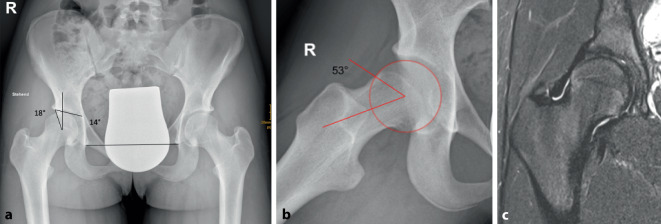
Die Cam-Morphologie kann bereits mit 10 Jahren auftreten und ist bei skelettaler Unreife zum Teil knorpelig angelegt, weshalb MRT-Untersuchungen aussagekräftiger als Röntgenbilder sind [25]. Zahlreiche Studien belegen, dass die Entstehung in den Jahren vor Schluss der Wachstumsfuge stattfindet und nach Fugenschluss nicht weiter fortschreitet [1, 19, 27]. Die häufigste Lokalisation ist anterosuperior am Schenkelhals, oft sind beide Hüften betroffen. Wie bereits weiter oben erwähnt, kommt es radiologisch zum Bild der epiphysären Extension (Abb. 2a,c), die prädiktiv für die Entwicklung einer Cam-Morphologie ist [20, 28]. Eine mögliche Erklärung ist, dass es sich um eine natürliche Reaktion auf Scherkräfte und juxtaphysäre Mikrotraumata handelt, die zu einem sogenannten Cupping-Phänomen führen, das wiederum einen Stabilisierungsversuch darstellt. Dies läuft parallel zum Verlust des epiphysären Tuberkels, der eine Rolle für die Stabilität der Epiphyse spielt, ab [15]. Die Quantifizierung der Cam-Morphologie erfolgt durch Messung des Alpha-Winkels nach Nötzli auf Röntgenbildern (Abb. 2b,d), CT oder MRT [22]. Die Cam-Morphologie muss nicht zwingend symptomatisch werden. Es ist weiterhin nicht geklärt, welche Patienten mit Cam-Morphologie Hüftschmerzen entwickeln. Eine herabgesetzte Innenrotation wurde als Risikofaktor beschrieben [11]. Ein niedriger Tönnis-Winkel, sowie eine azetabuläre Retroversion dürften ebenfalls ungünstige Faktoren darstellen [16]. Prospektive Studien zur endgültigen Definition von Risikofaktoren für die Entwicklung der Koxarthrose fehlen [26].
